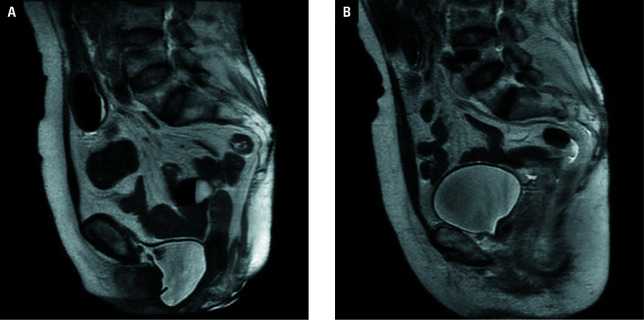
A – preoperative pelvic magnetic resonance imaging (MRI; Valsalva maneuver); B – 6-month postoperative pelvic MRI (Valsalva maneuver)
